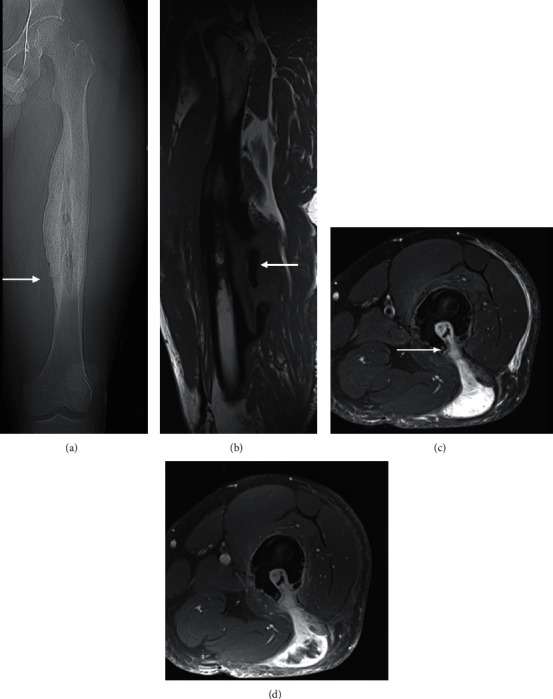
A 30-year-old systemically well man with chronic osteomyelitis who initially presented with an enlarging mass in the posterior thigh. The planning CT (a) showed femoral shaft sclerosis, periosteal thickening, and cortical expansion which is worse medially. A lucent tract at the inferior border of the abnormal bone extends from the medulla to the cortex, consistent with a sinus tract (arrow). Sagittal T1 (b) demonstrating the extent of the osteomyelitis with sequestrum posteroinferiorly (arrow). Axial PDFS (c) and T1FS postcontrast (d) MRI shows the new bone formation or involucrum attempting to envelop the infection. A cloaca or sinus tract involving the posterolateral thigh discharges into the subcutaneous plane, accounting for the presentation (C arrow).
